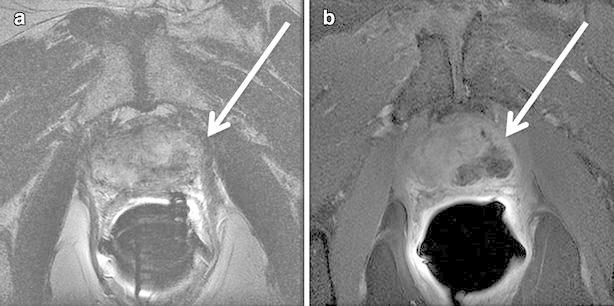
Typical findings on follow-up MRI 3 weeks after IRE of the prostate. From one patient, a the treatment zone appears as heterogeneous hyperintense region (*arrow*) with regions of low signal intensity. b The lesion (*arrow*) is easily visualized on contrast enhanced T1w imaging
